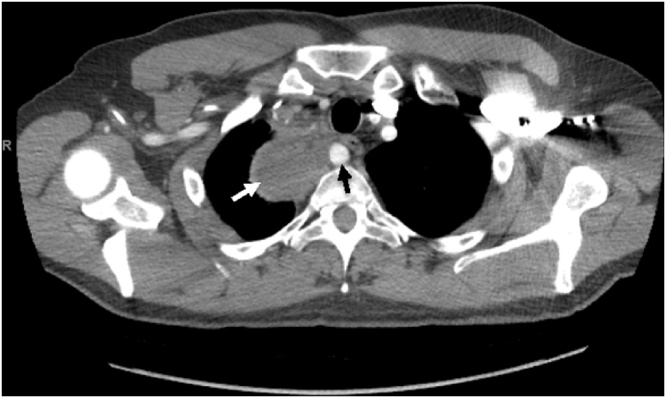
CT demonstrating retroesophageal SCA (black arrow) adjacent to esophageal leiomyosarcoma (white arrow).
